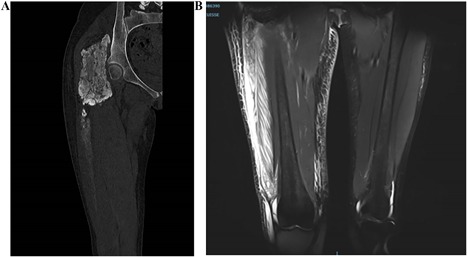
Imaging of the disease flare of December 2017. (*A*) CT scan, bone window: calcic mass in gluteal muscle, erosion of iliac bone for 160 mm with bone condensation, disintegration at the lower pole, extending on more than 200 mm with liquid collection under quadricipital superficial aponeurosis. (*B*) MRI in STIR sequence: collection 300 × 75 × 20 mm with heterogenic content in high STIR signal, with compounds of low STIR signal corresponding with calcic liquid. Muscle swelling; no necrosis.
